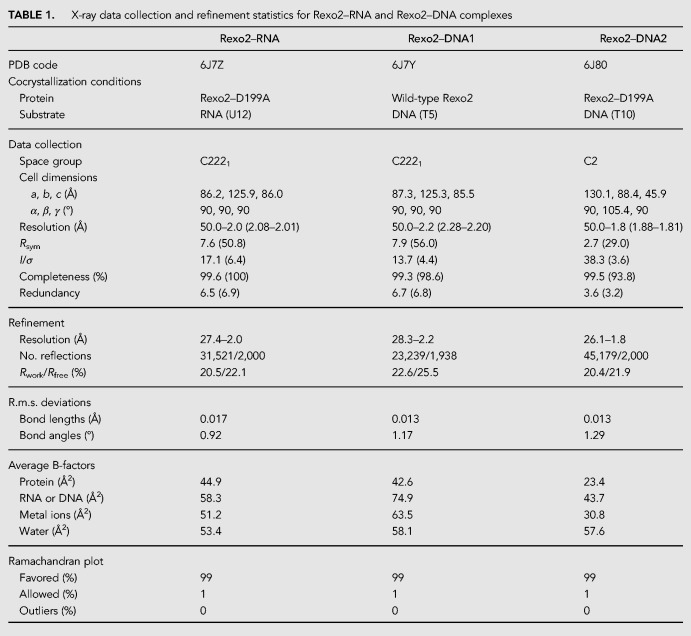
X-ray data collection and refinement statistics for Rexo2–RNA and Rexo2–DNA complexes
